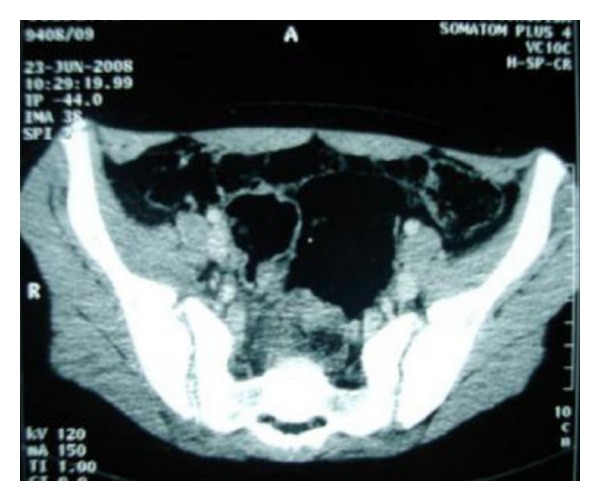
CT of the pelvis showed signs of right sacroiliitis with infiltration and microabscess of the soft tissue, surrounding the joint with erosions of the subchondral iliac bone.
